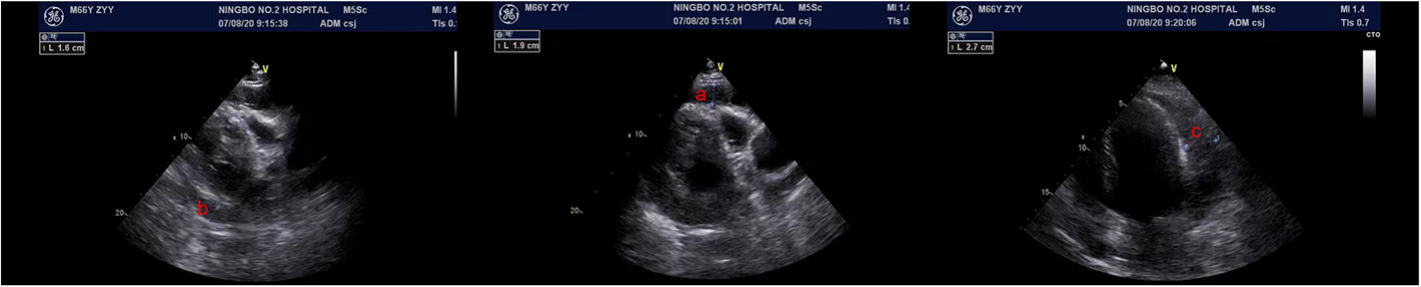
The echocardiography on August 7th shows massive pericardial hemorrhage. a, b and c indicate the thickness of the effusion of the anterior, posterior and left lateral pericardium cavity was 19 mm, 17 mm, and 27 mm, respectively
